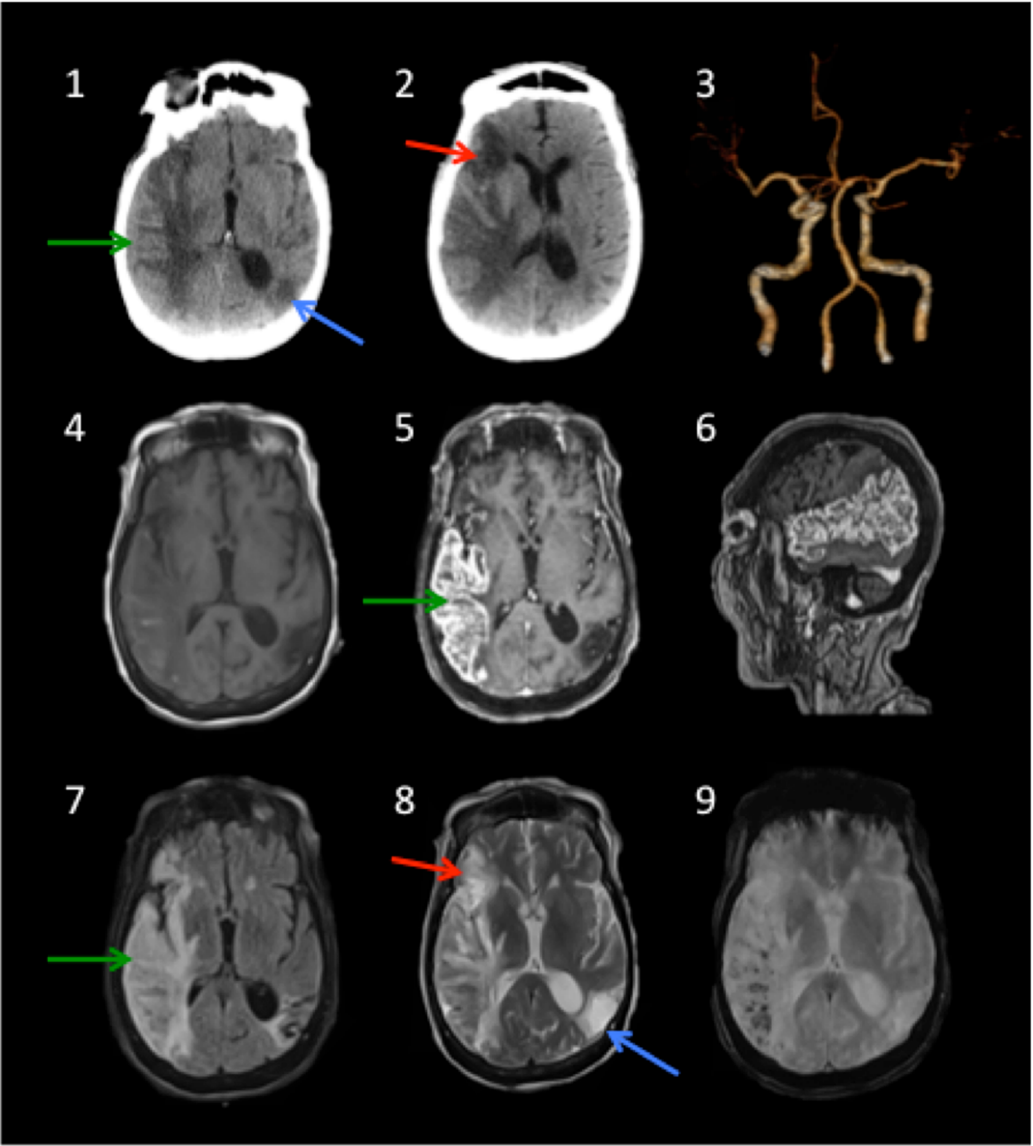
Strokes and concurrent subacute inflammatory necrotizing response. An 84-year-old SARS-CoV-2-positive female presented solely with rapidly progressive encephalopathy and neurological decline associated with mild left sided hemiparesis and left sided-visuospatial neglect. There were no concurrent respiratory or any other systemic symptoms. Serological findings were remarkable for CRP of 5.89 mg/dl, ferritin of 383 ng/dl, and D-Dimer of 852 ng/dl, and positive anticardiolipin IgM antibodies of 32.CSF analysis revealed elevated protein (153 mg/dl), normal glucose, and a cell count of three nucleated cells. Computed tomography (CT) scan (1, 2) shows prominent vasogenic edema nearly involving the entire right temporal lobe (green arrow), watershed subacute-chronic ischemic infarction of the right anterior frontal (red arrow), and old cystic encephalomalacia of the left parietal (blue arrow). 3D CT angiogram (3) shows normal flow through the right middle cerebral artery without evidence of large vessel occlusion or high grade stenosis. Axial T1-(4) and T1-weighted post-gadolinium [axial (5) and sagittal (6)] MR images show diffuse gyral cortical enhancement with subcortical areas of necrosis involving right temporal lobe (green arrow). Axial FLAIR (7) and axial T2W (8) MR images reveal signal hyperintensities with gyral swelling nearly involving the entire right temporal lobe and insula (green arrow) associated with petechial hemorrhage, best seen on the gradient echo image (9). In addition, there are subacute-chronic infarct of the right anterior lateral frontal lobe (red arrow) and a wedge-shaped old cystic encephalomalacia of the left parietal lobe (blue arrow). Following treatment with tocilizumab 560 mg (4 mg/kg), the patient exhibited significant improvement of the mental status and left hemiparesis and was subsequently discharged home. Hypercoagulopathy is the likely etiology of the right frontal and left parietal strokes. The appearance of the concurrent right temporal lobe and insula abnormalities is highly suggestive of an independent subacute inflammatory-necrotizing, rather than ischemic, process. This is supported by the findings of completely patent right middle cerebral artery, diffuse gyral swelling and enhancement with concurrent prominent subcortical vasogenic edema and necrosis, and significantly elevated CSF protein. Although the precise mechanisms underlying vasogenic edema and necrosis remain unclear, we suggest that localized hyper-inflammation provoked by exuberant innate immune response (CNS cytokine response) might be pathogenetically relevant. Direct viral neuroinvasion and involvement of the neurovascular endothelial cells were subsequently excluded by the brain biopsy from the right temporal necrotizing lesion
